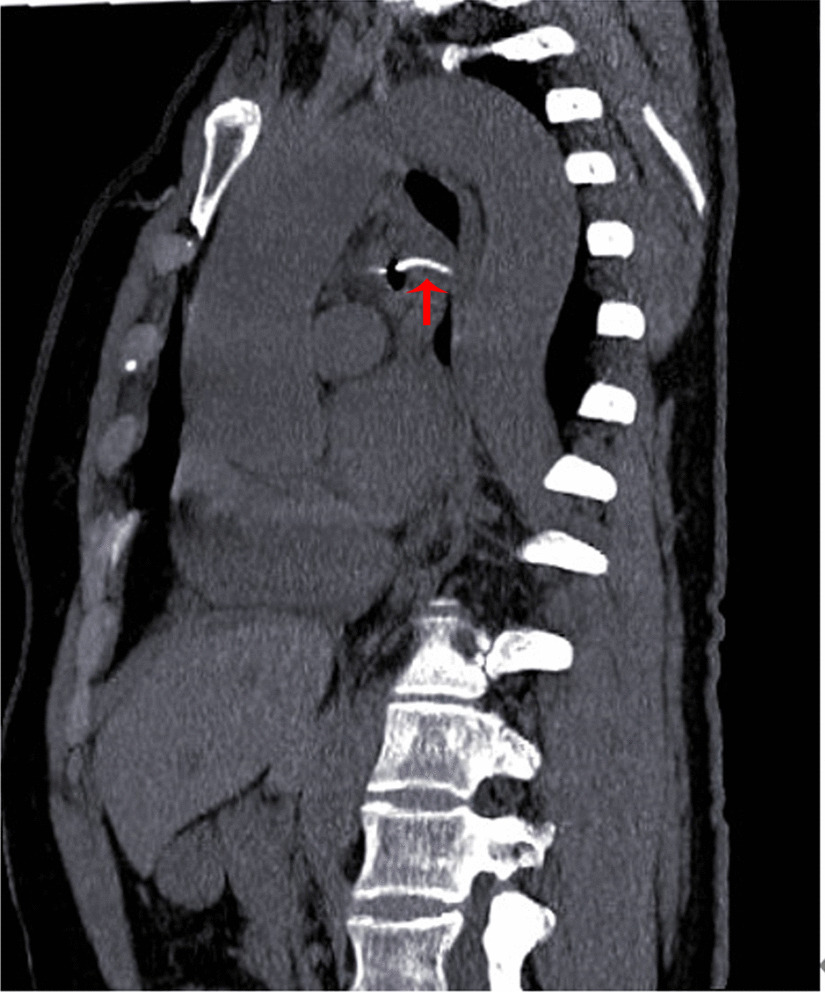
CT sagittal reconstruction image of the chest. The figure shows that a slender fish bone traverses the esophagus, mediastinum, and left main bronchus
